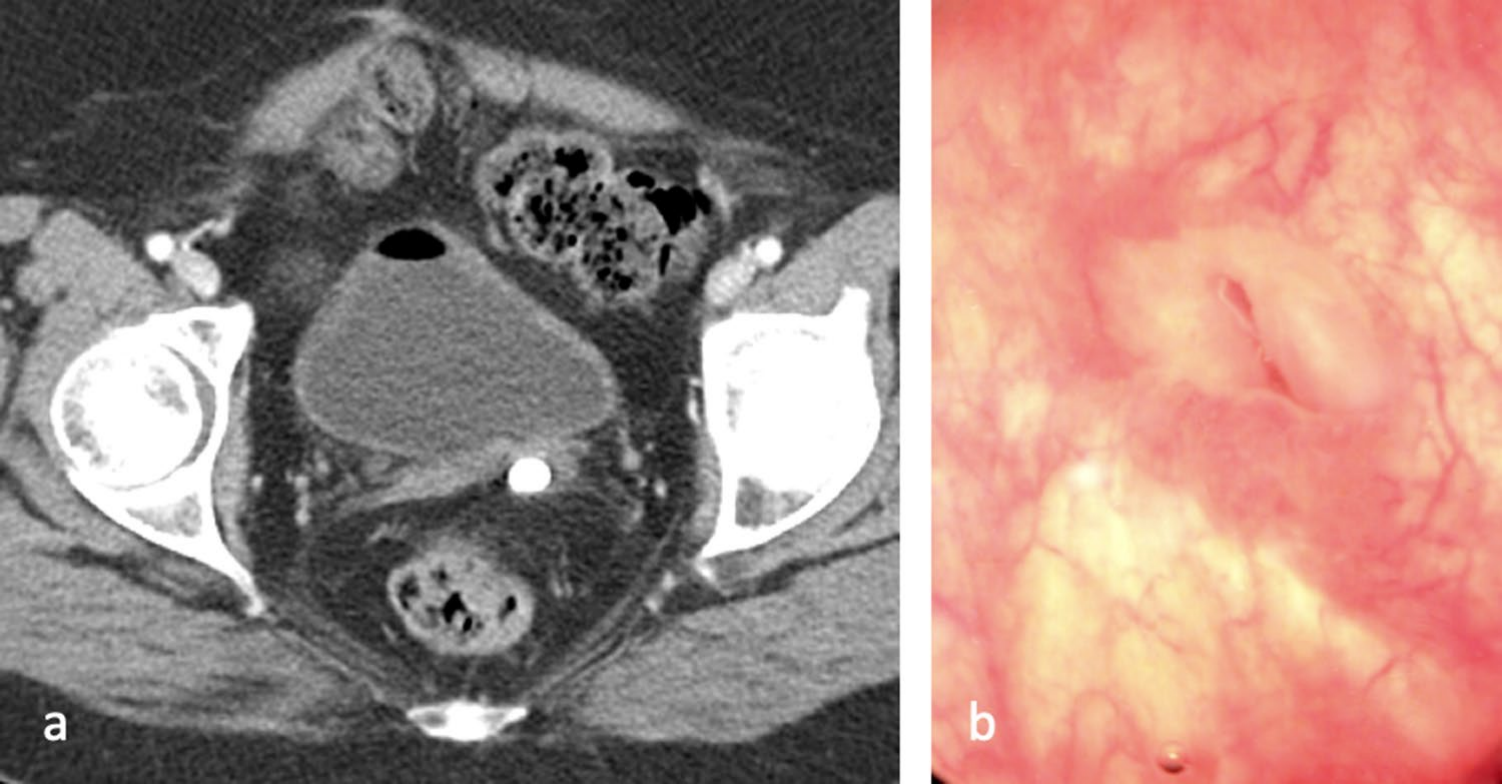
50-year-old female with history of hysterectomy complicated by vesicovaginal fistula status post repair presenting with dysuria. Axial CT through the pelvis a demonstrates a bladder stone posterior to the bladder and adjacent to the left vaginal cuff. Cystoscopy b demonstrates a defect in the bladder wall related to a sinus tract from the previous vesicovaginal fistula repair. Surgery was later performed and the stone was removed from the bladder sinus tract
